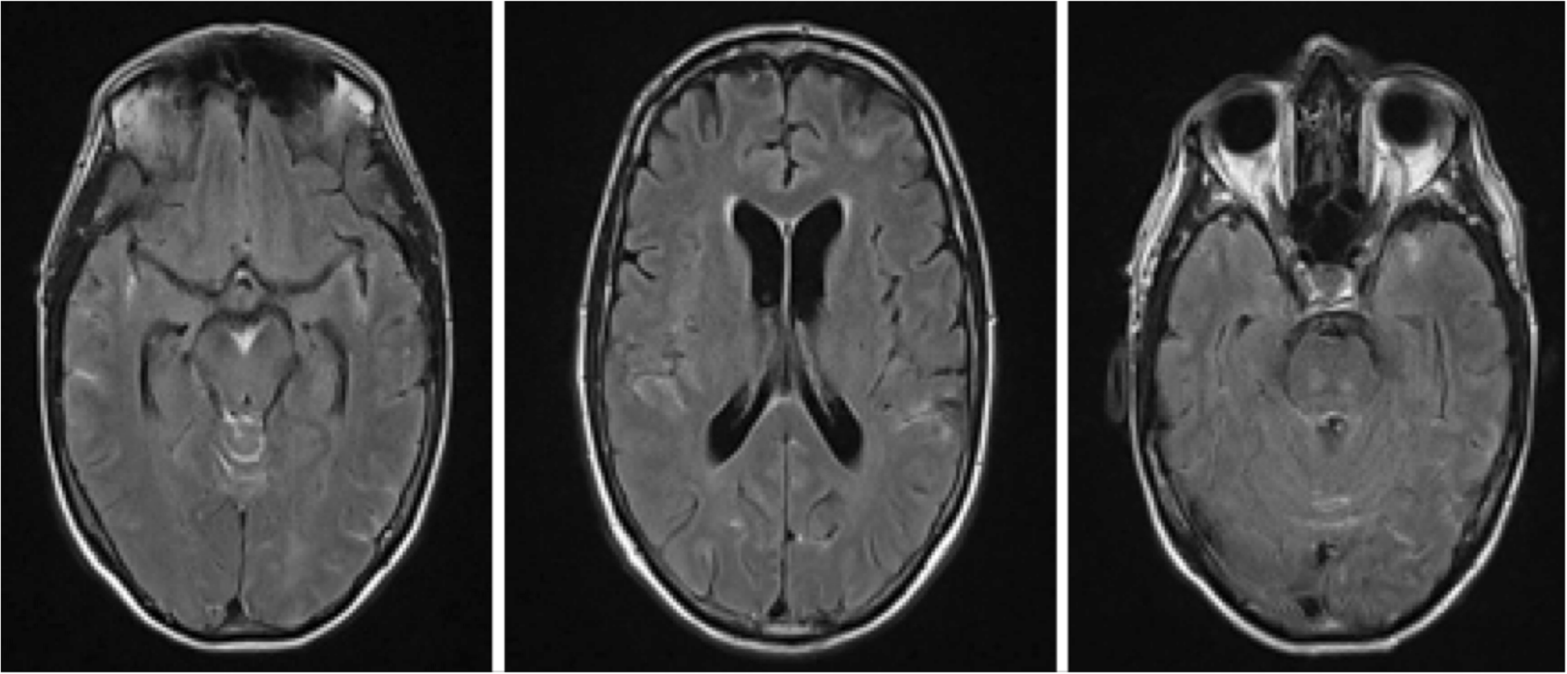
Magnetic resonance images of the brain showing diffuse subarachnoid fluid-attenuated inversion recovery (FLAIR) hyperintensity concerning for blood within the parafalcine, left frontal, bilateral occipital and bilateral temporal sulcal spaces, Sylvian fissures and the basal cistern. It also showed a focus of cortical/subcortical high signal in the left superior parietal lobule. No meningeal or parenchymal enhancement was demonstrated
